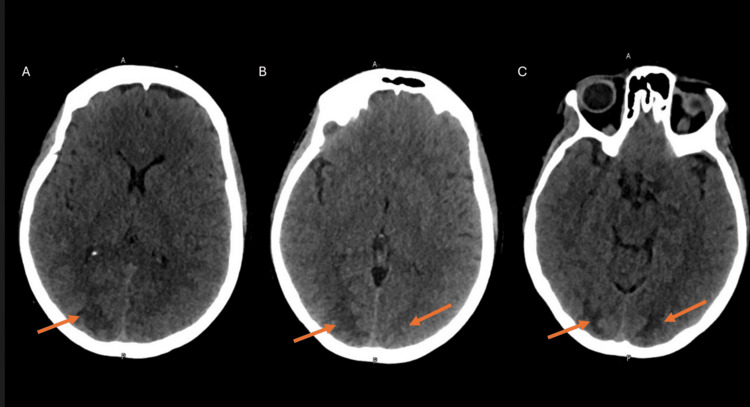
Noncontrast CT scan of the head (axial views; A-C) demonstrating bilateral, predominantly subcortical, near-symmetrical ill-defined hypodensities in the parieto-occipital regions (arrows), consistent with possible PRES PRES, posterior reversible encephalopathy syndrome
